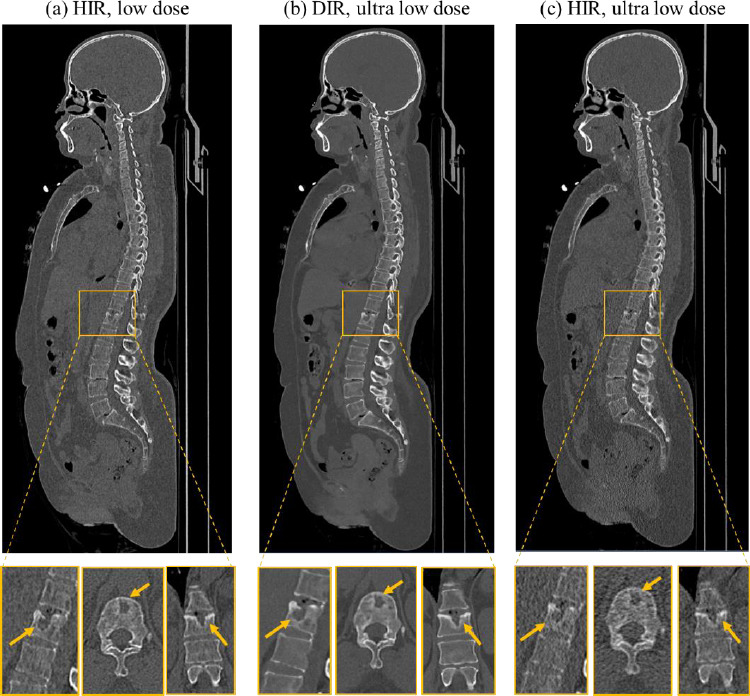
Whole-body CT obtained from a 54-year-old woman with multiple myeloma. Images were acquired at low dose (6.57 mGy) and ultra-low dose (1.39 mGy). The low-dose image was reconstructed with HIR (a), and the ultra-low-dose image was reconstructed with DIR (b) and HIR (c). Detailed graphs of the osteolytic lesion, in the sagittal, axial, and coronal views, are displayed at the bottom. At the ultra-low-dose level, DIR provides optimized visualization of osteolytic lesions as compared with HIR, whereas the vertebral body may appear over-smoothed.
